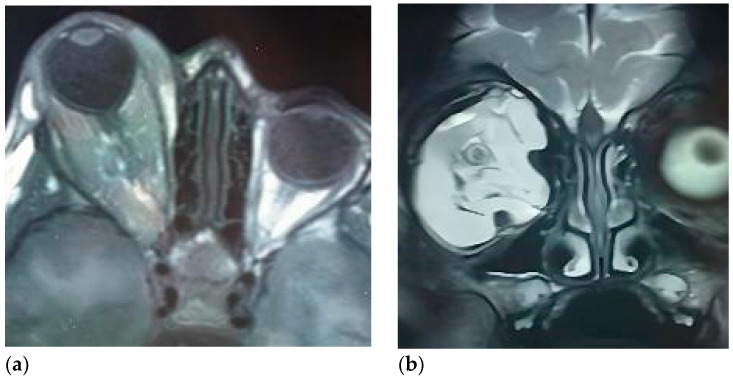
MRI image of the lymphatic malformation affecting almost 360° of the orbital compartment. (a) axial view; (b) coronal view.
